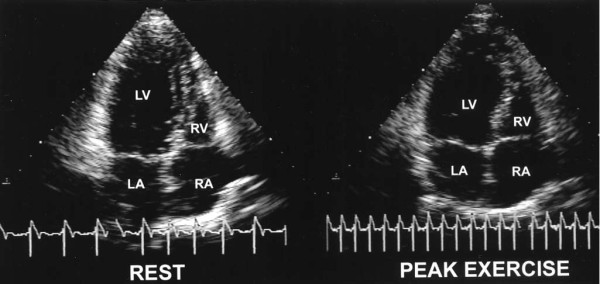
Enlarged left ventricular cavity after peak exercise. Exercise echocardiogram obtained from an apical four-chamber view of the heart in a 73-year-old woman with a hypertensive response to exercise. An end-systolic image taken immediately after peak exercise (right) shows an enlarged left ventricular (LV) cavity compared with image at rest (left). LA: left atrium; RA: right atrium; RV: right ventricle [39].
